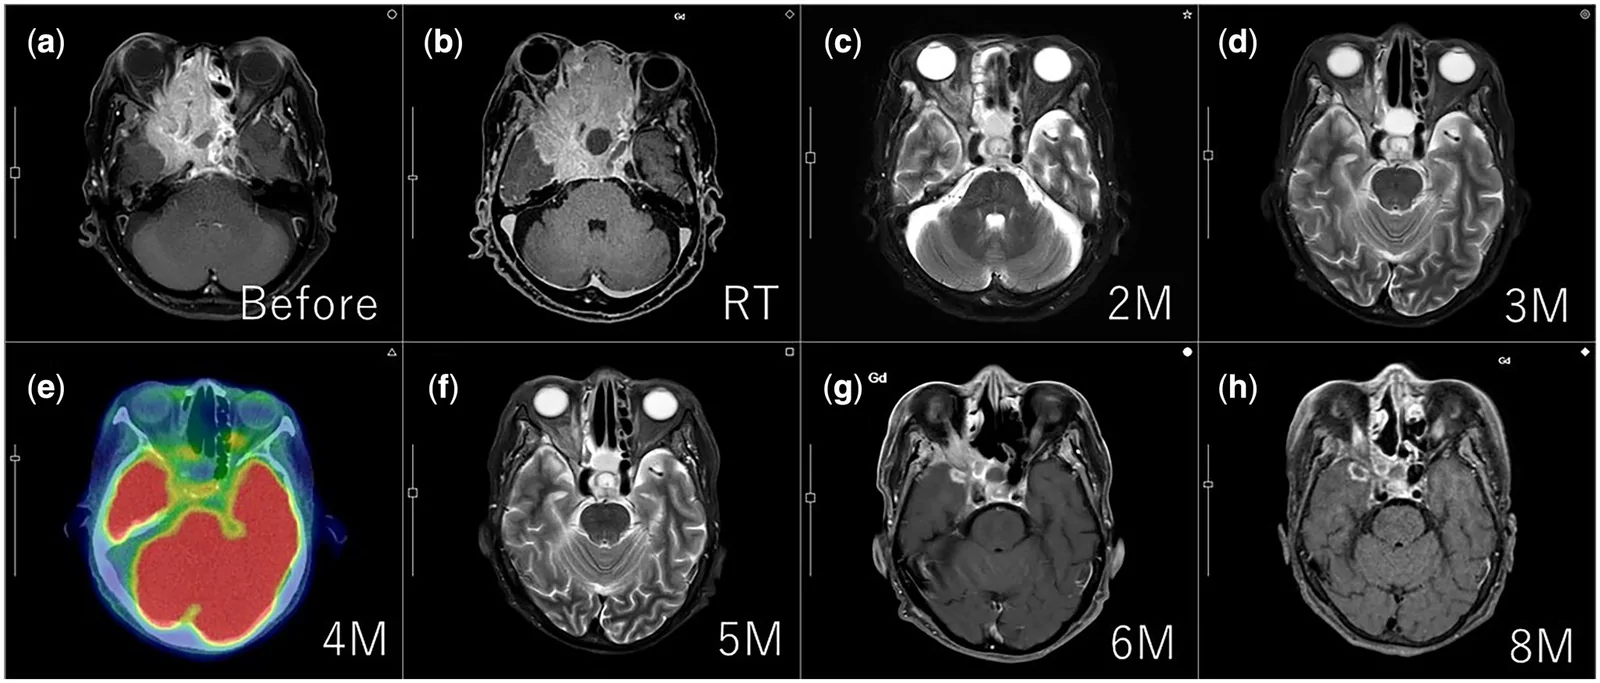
Changes over time. MRI images from A to H (only (E) is fluorodeoxyglucose positron emission tomography). (A) “Before” image at the time of referral to our department. (B) “RT” image at the start of radiation therapy. (C) and onwards: the number of months since treatment is indicated in M (months). (D, E) FDG-PET and (F) No signs of recurrence. (G) Primary lesion remained unchanged and small brain metastases (right temporal lobe) observed. (H) Primary lesion remained unchanged, but brain metastases tended to grow.
